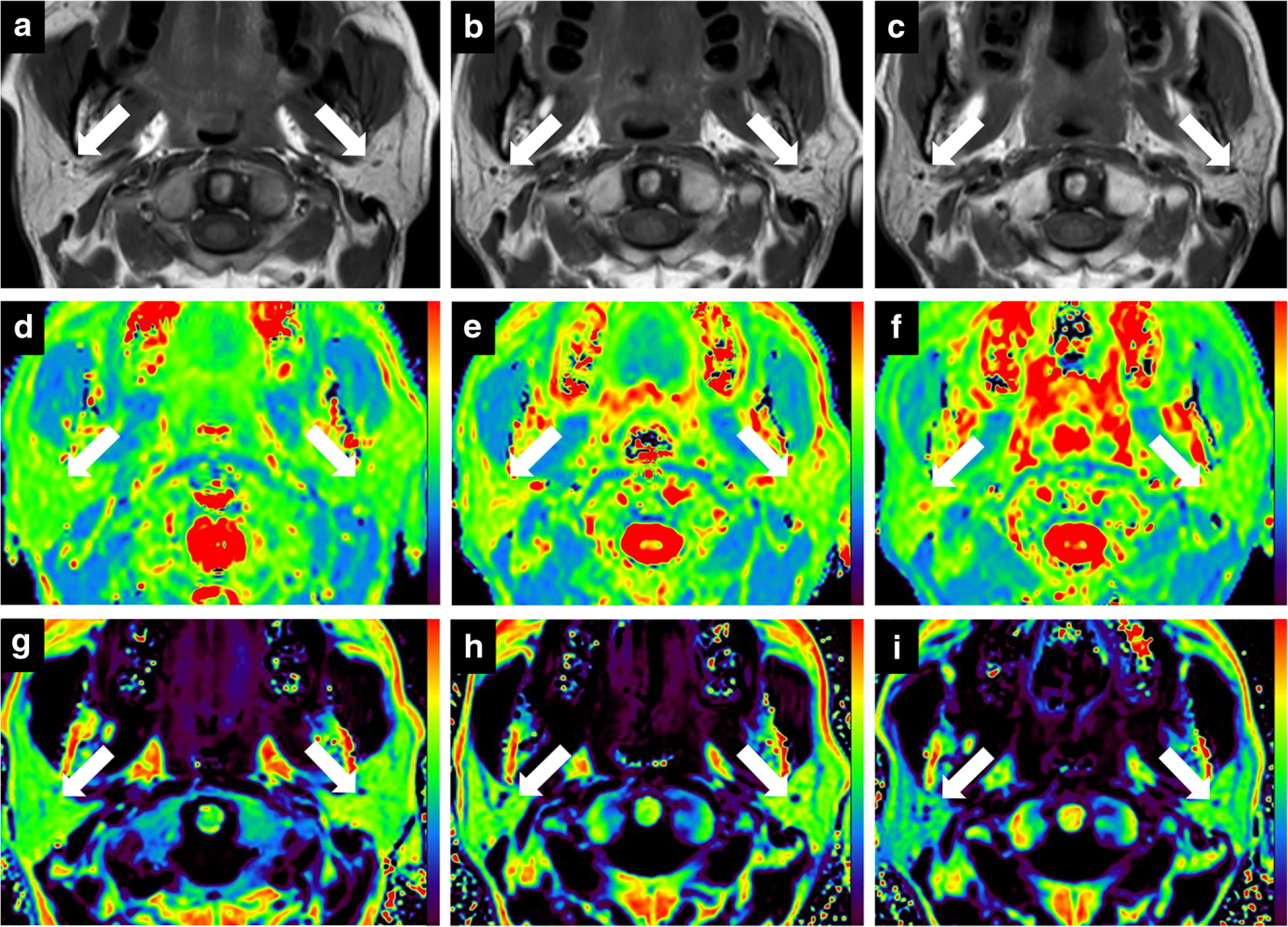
Dynamic changes of T1-weighted images (a-c), T2 maps (d-f), and fat fraction (FF) (g-i) maps of bilateral parotid glands (white arrows) in one patient with nasopharyngeal carcinoma (NPC) within 2 weeks before radiotherapy (pre-RT), 5 weeks after the beginning of radiotherapy (mid-RT), and 4 weeks after radiotherapy (post-RT), respectively. T1-weighted images show that right and left parotid volume are 30.1 cm^3^ and 30.2 cm^3^ (pre-RT, a), 21.9 cm^3^ and 20.1 cm^3^ (mid-RT, b), and 23.2 cm^3^ and 20.2 cm^3^ (post-RT, c), respectively. T2 maps show that right and left parotid T2 values are 70.9 msec and 65.8 msec (pre-RT, d), 75.4 msec and 75.1 msec (mid-RT, e), and 75.8 msec and 72.2 msec (post-RT, f), respectively. FF maps show that right and left parotid FF values are 54.9% and 57.4% (pre-RT, g), 59.0% and 57.3% (mid-RT, h), and 45.9% and 50.4% (post-RT, i), respectively
